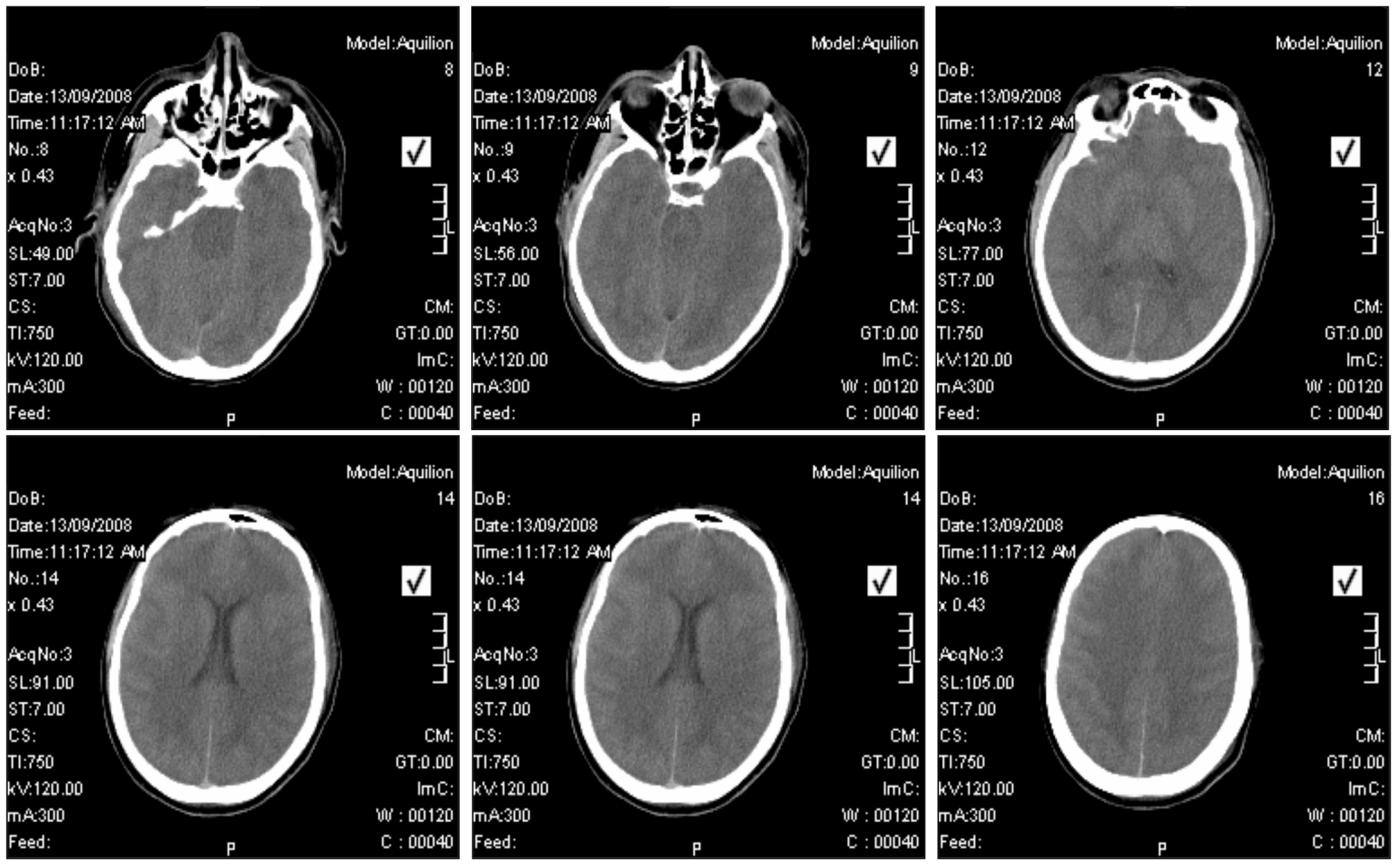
CT scan of the brain of Patient 5 showing cerebral edema on illness day 12.
